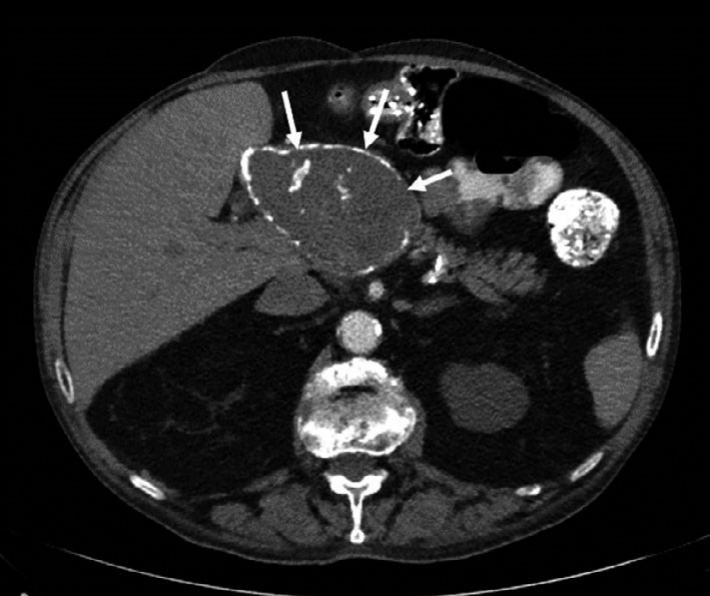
Computed tomography angiography at 24 months depicted spontaneous thrombosis of the common hepatic artery aneurysm (arrows)
